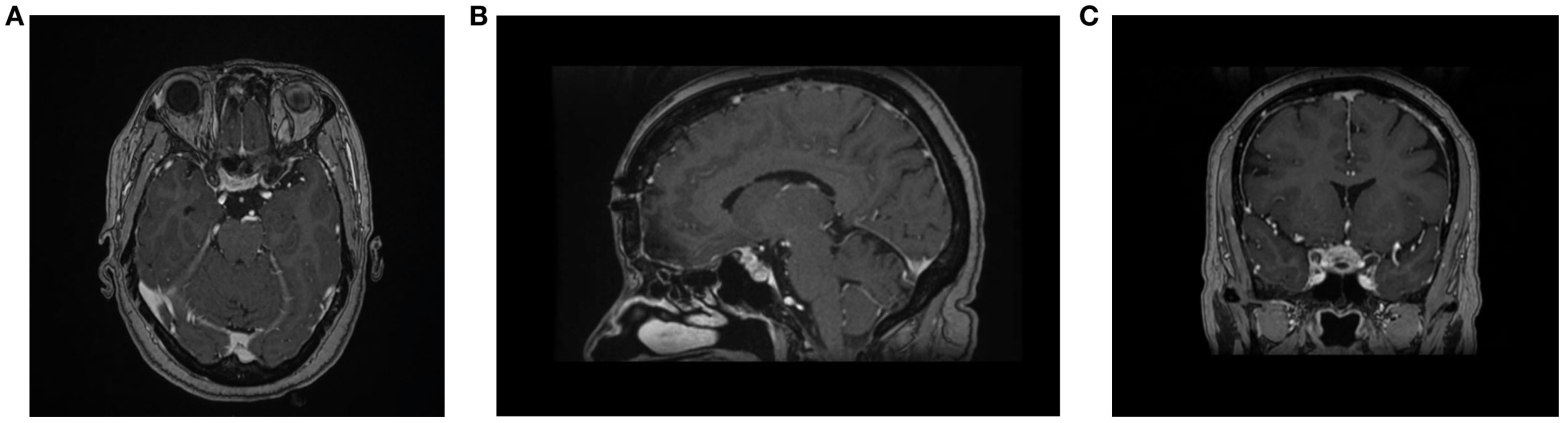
(A) Axial, (B) coronal, and (C) sagittal contrasted MRI showing a small (0.8x0.8 cm) tuberculum sellae meningioma that extends into the right optic canal.
